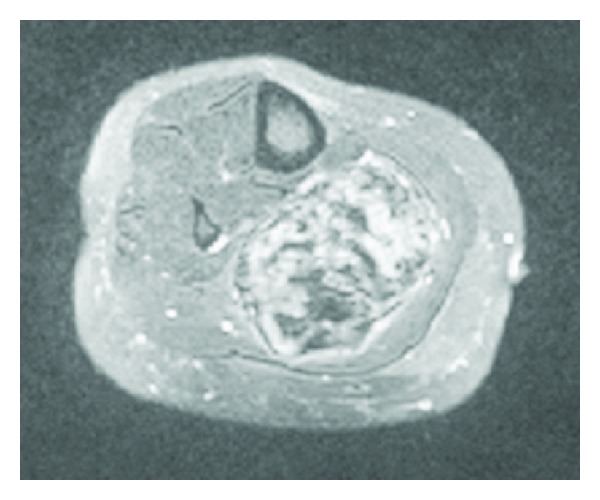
T2-weighted axial MRI of right lower leg showing heterogenous mass.
